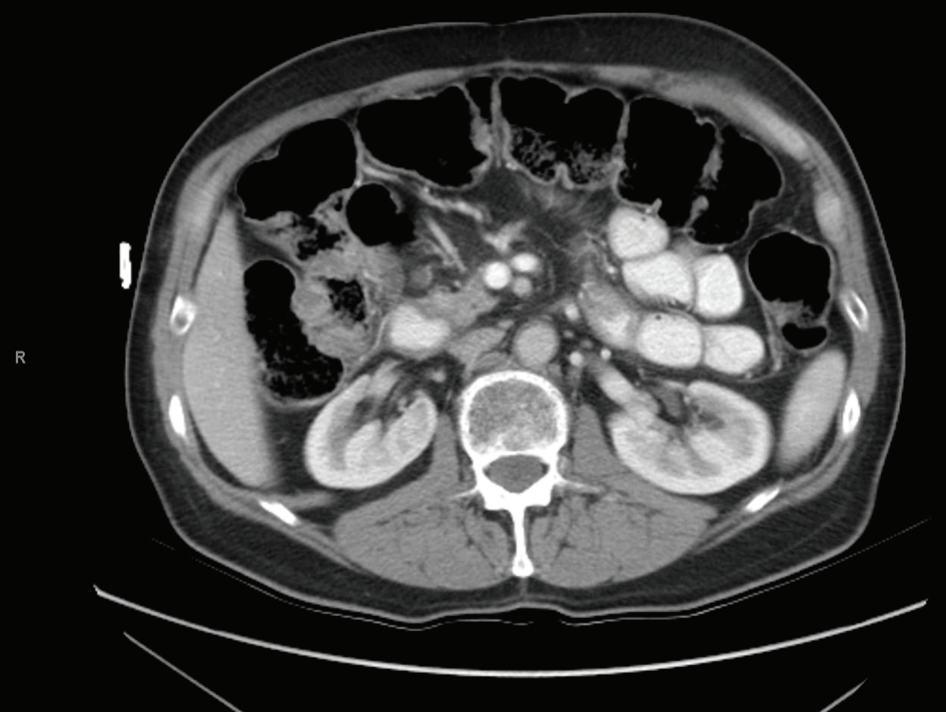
CT scan 3 years after aborted chemoradiation therapy.
